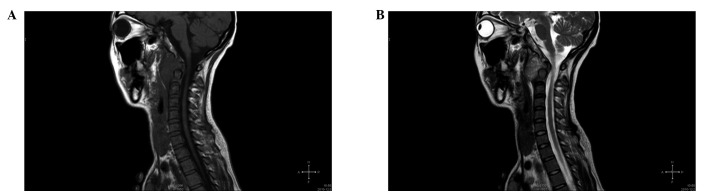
Female, 31-year-old patient with severe neck pain. Sagittal MRI scans revealed an extremely large chordoma centered at C1 and extending to the anterior aspects of C2. (A) T1-weighted and (B) T2-weighted sagittal MRI. MRI, magnetic resonance imaging.
